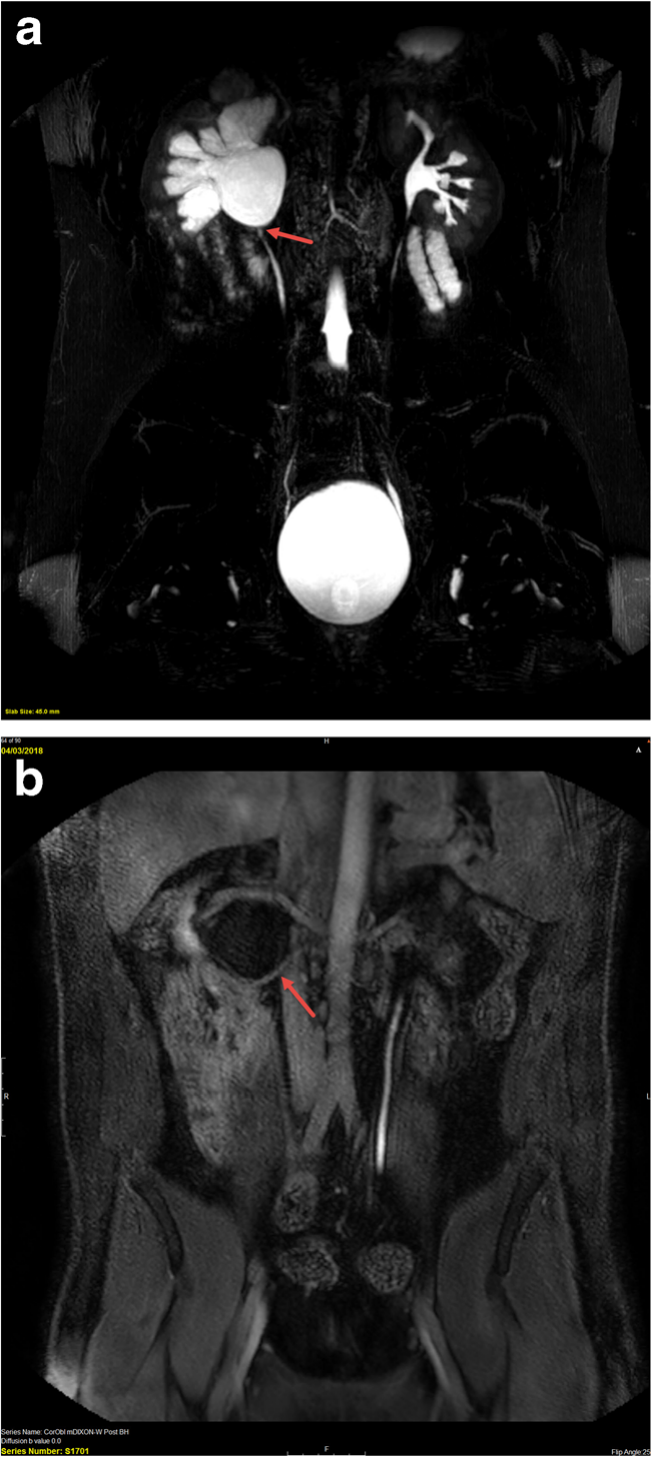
Sixteen-year-old with right flank pain and right hydronephrosis discovered on renal US. a 3D T2-weighted image shows moderate right pelvocaliectasis with an abrupt transition to a normal caliber ureter (arrow) consistent with a ureteropelvic junction obstruction. b Post-contrast excretory phase image shows a crossing accessory renal artery (arrow) supplying the lower pole of the right kidney. The renal pelvis is dilated proximal to the crossing vessel, and the ureter distal to it is normal in caliber. There is no visible excreted contrast material in the right renal collecting system, while contrast material is seen in a normal caliber left ureter
